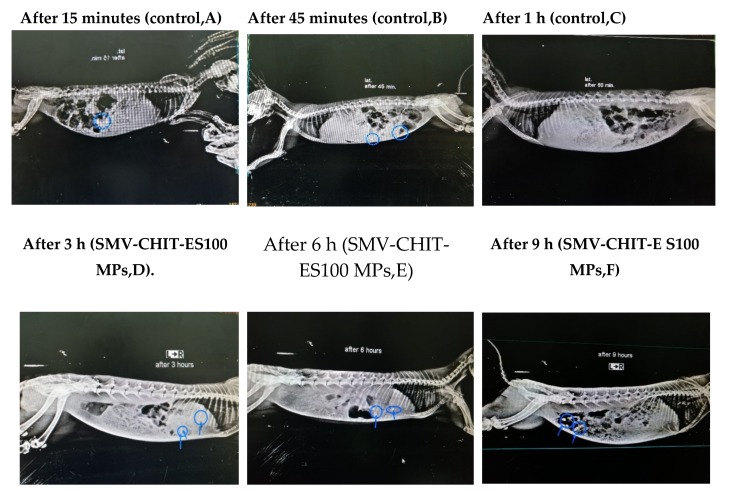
X-ray photographs of (control) after 15 (A), 45 (B) and 60 min (C) and for SMV-CH-E S100 MPs after 3 (D), 6 (E) and 9 h (F).
